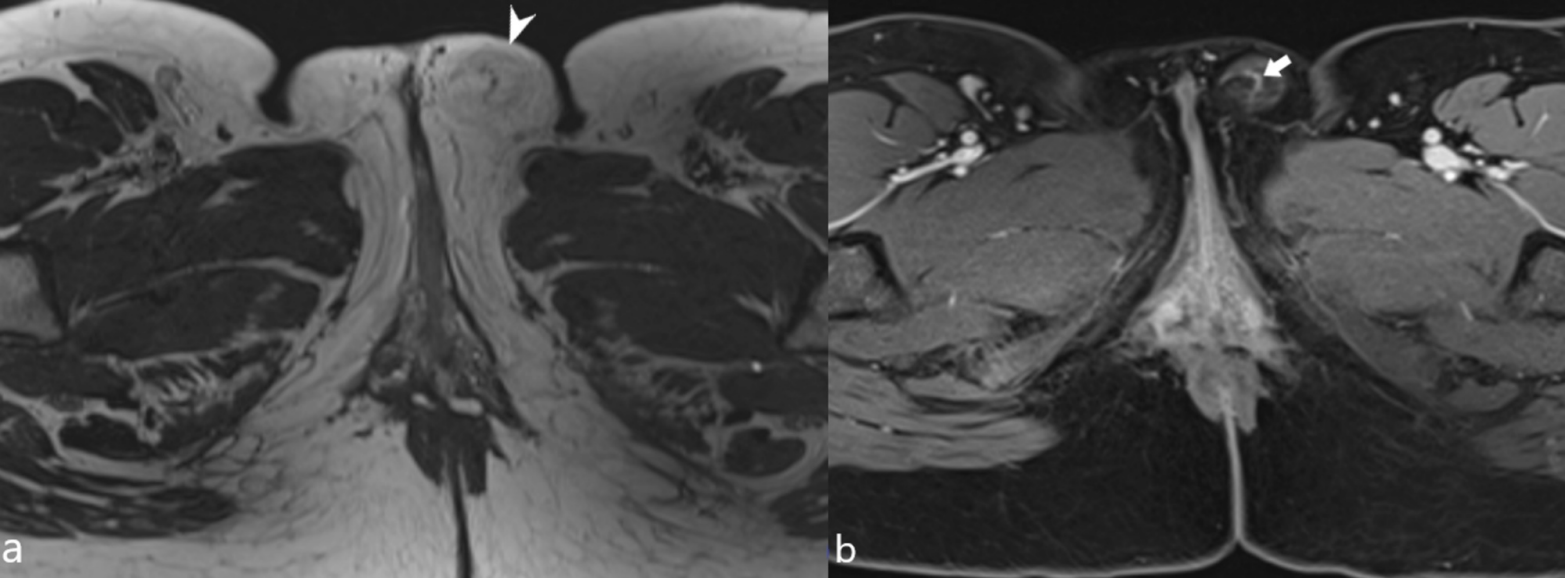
MRI images of a vulvar hibernoma (a) Pre-contrast T1-weighted MRI shows a circumscribed, lipomatous mass (white arrowhead) within the left vulva with mild T1 hypointensity compared to subcutaneous fat. (b) Post-contrast, fat saturation, T1-weighted MRI shows heterogeneous enhancement with a prominent vessel (white arrow) coursing through the central portion of the tumor.
